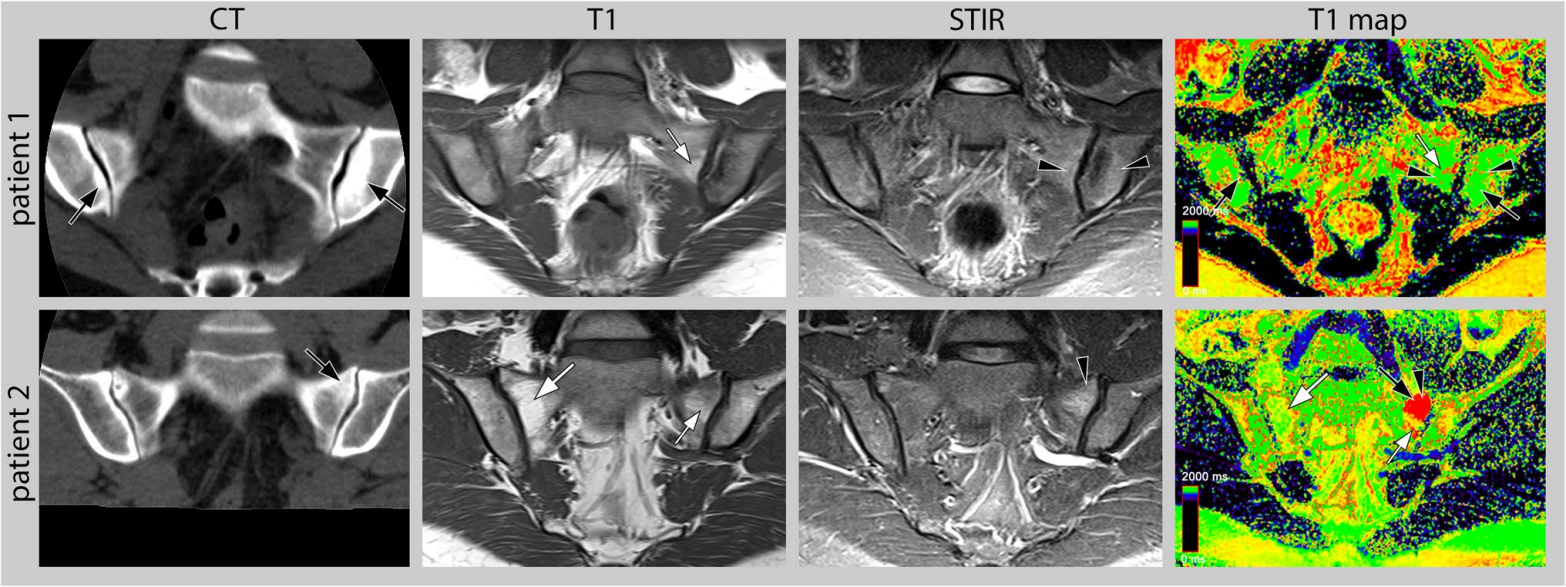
Mixed lesions. Presented are mixed lesions in CT (window level / window width: 240/730) and MRI (T1, STIR, and T1 map). Patient 1 (top row) shows a lesion with sclerosis (black arrow) and bone marrow oedema (black arrowhead) in the left ileum and a lesion with bone marrow oedema and fat (white arrow) in the left sacrum that are not visible in the T1 map. However, the small sclerotic lesions in the right ileum are detectable. Patient 2 (bottom row) shows a mixed lesions of sclerosis (black arrow), fat (white arrow) and bone marrow oedema (black arrowhead) that shows artificially increased values in the T1 map (black arrowhead and arrow). The fat lesion of the right sacrum is displayed normally (white arrow)
